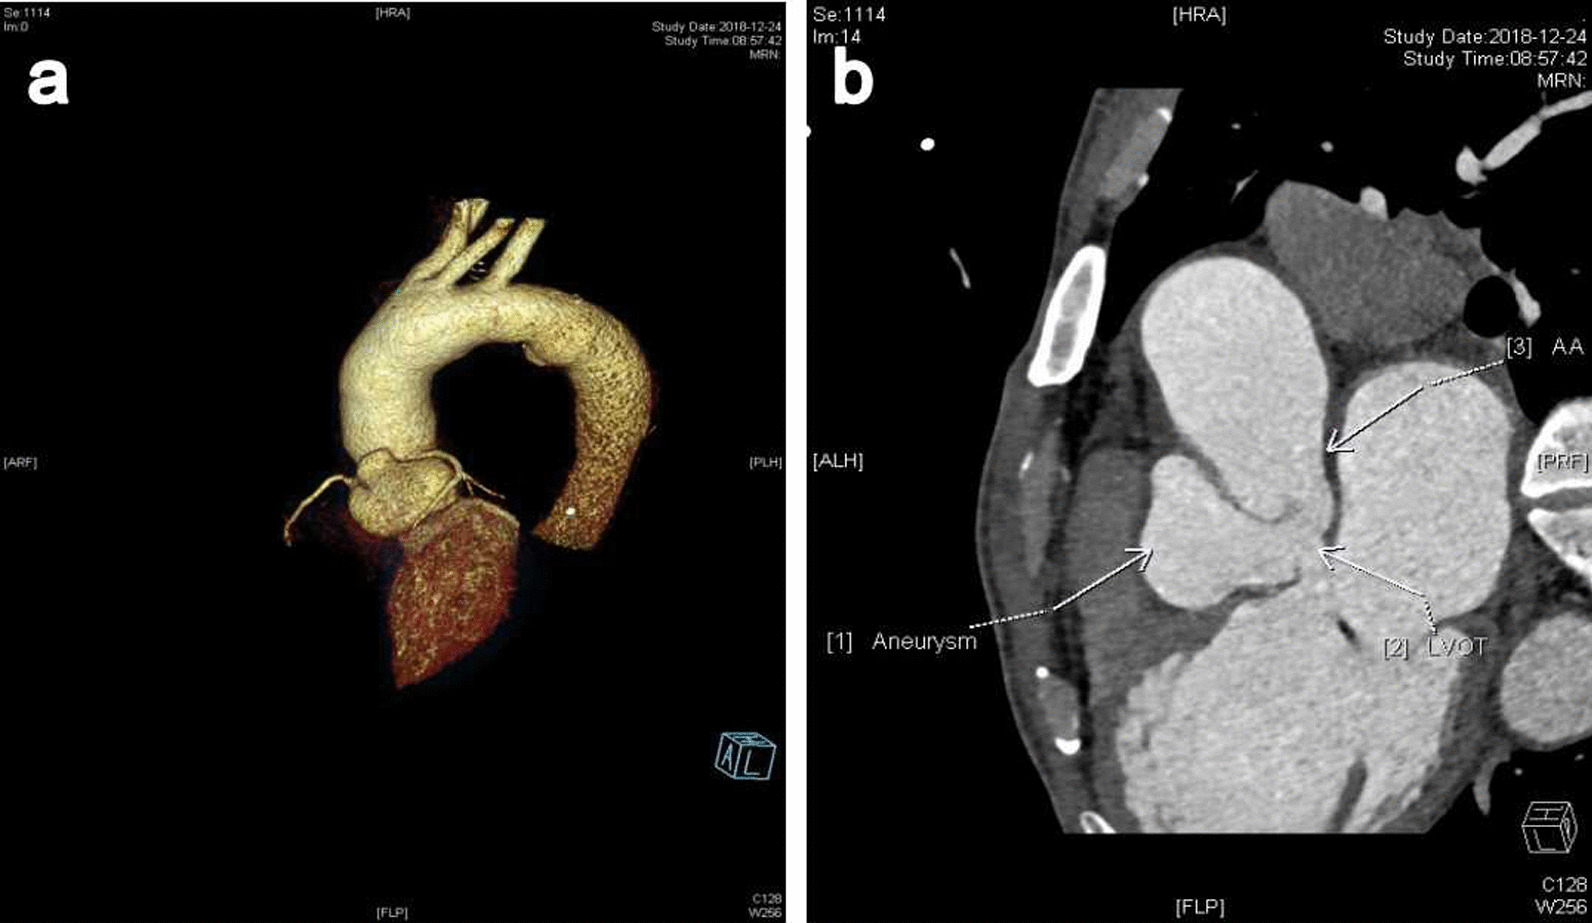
Preoperative examination. a Three-dimensional construction of cardiac computed tomography angiogram. b An aneurysmal ALVT was demonstrated with an oval entrance in the tubular aorta above the the right-coronary sinutubular ridge, draining into the LVOT. *ALVT* aorto-left ventricular tunnel, *LVOT* left ventricular outflow tract
